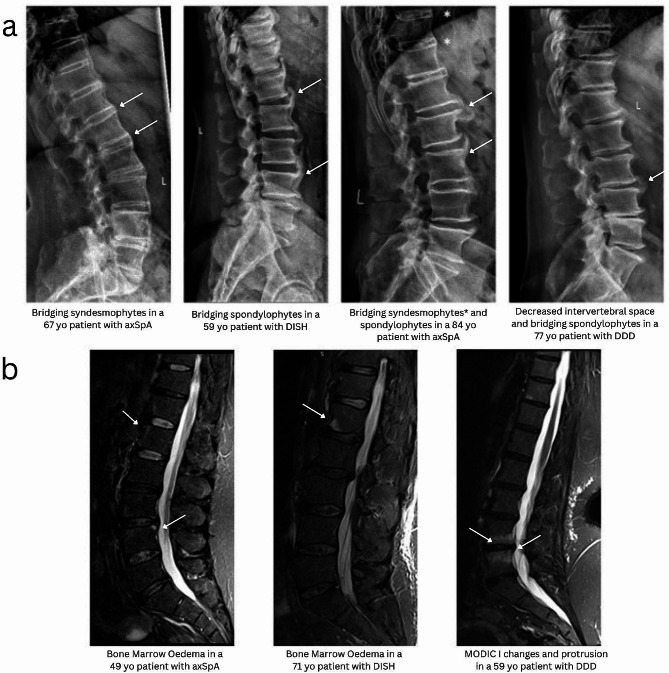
Sample imaging of spinal lesions: a Sample CRs of spinal lesions in patients with axSpA, DISH, and DDD. b Sample MRIs of spinal lesions in patients with axSpA, DISH, and DDD. axSpA: axial Spondyloarthritis, CR: Conventional Radiography, DDD: Degenerative Disc Disease, DISH: Diffuse Idiopathic Skeletal Hyperostosis, MRI: Magnetic resonance Imaging. Characteristic lesions were indicated by arrows
